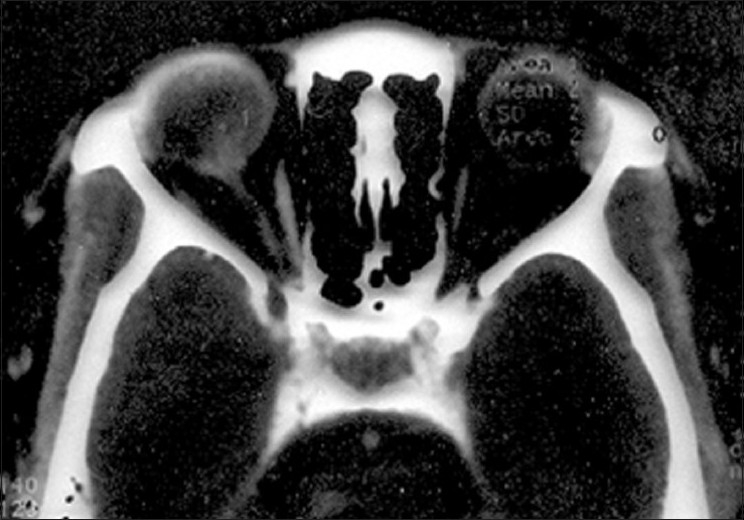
On contrast enhanced computed tomography (CECT) of orbit, a contrast enhanced mass at posterior pole with absence of calcification was reported
